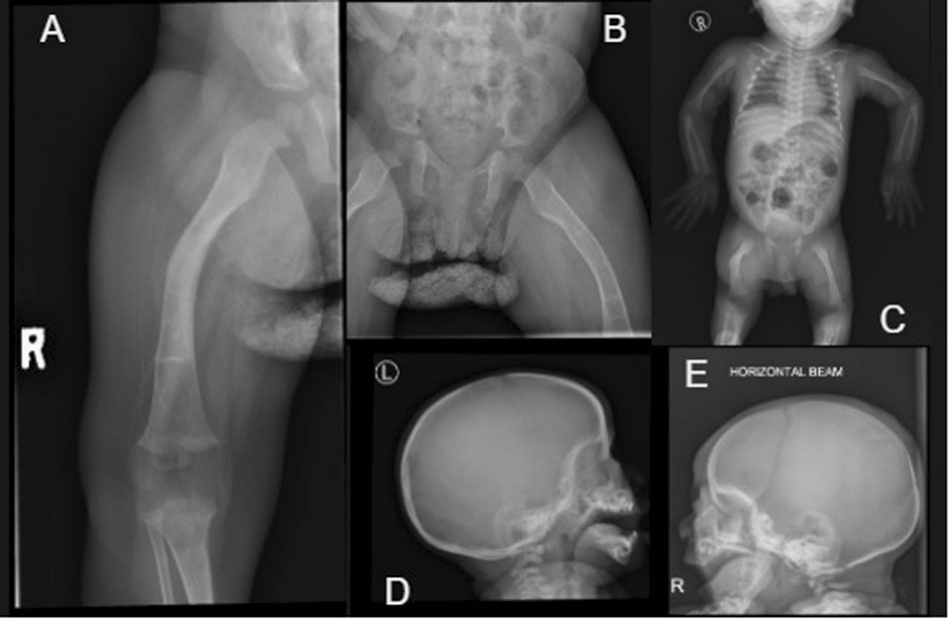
Radiographs of patient 13 (*FKBP10*). A Right femur deformity with osteopenia, metaphyseal flaring at the knee at age 13 months. B Right femur showing deformity, osteopenia at age 11 months. C Babygram at age 9 days showing generalised osteopenia and angulated right femur. D Lateral skull at 11 months showing Wormian bones. E Lateral skull at 9 days showing Wormian bones and patent coronal sutures.
